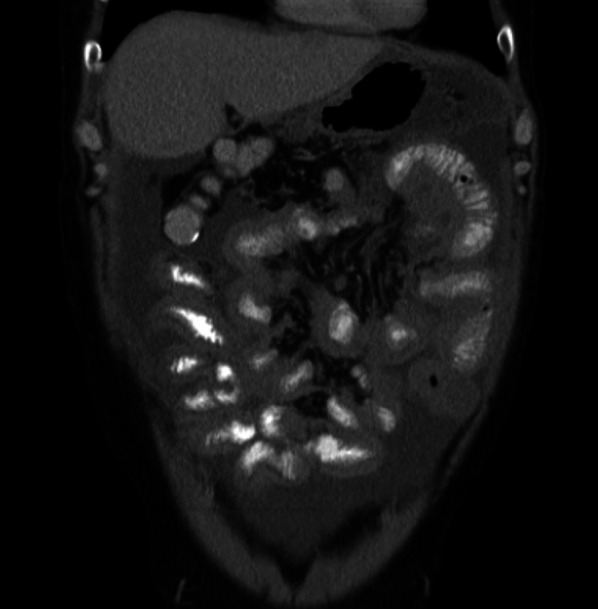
CT scan, coronal image of a patient with advanced epithelioid malignant peritoneal mesothelioma showing a rind of soft tissue around the small bowel due to diffuse thickening of the small bowel serosa or the visceral peritoneum
